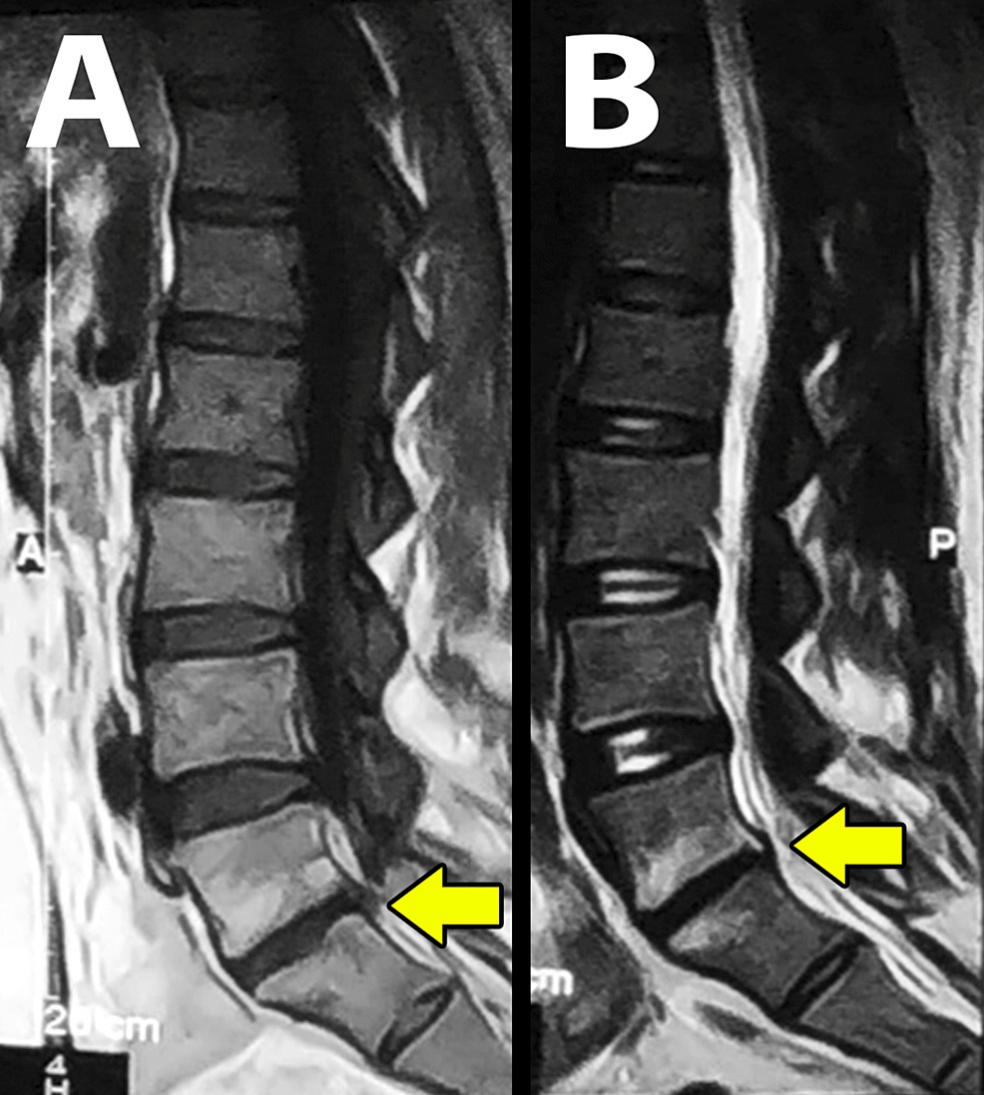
Follow-up MRI images. T1-weighted (A) and T2-weighted (B) MRI in the sagittal plane about one year after the treatment. The imaging showed relative L5-S1 disk space narrowing without inflammatory changes.
